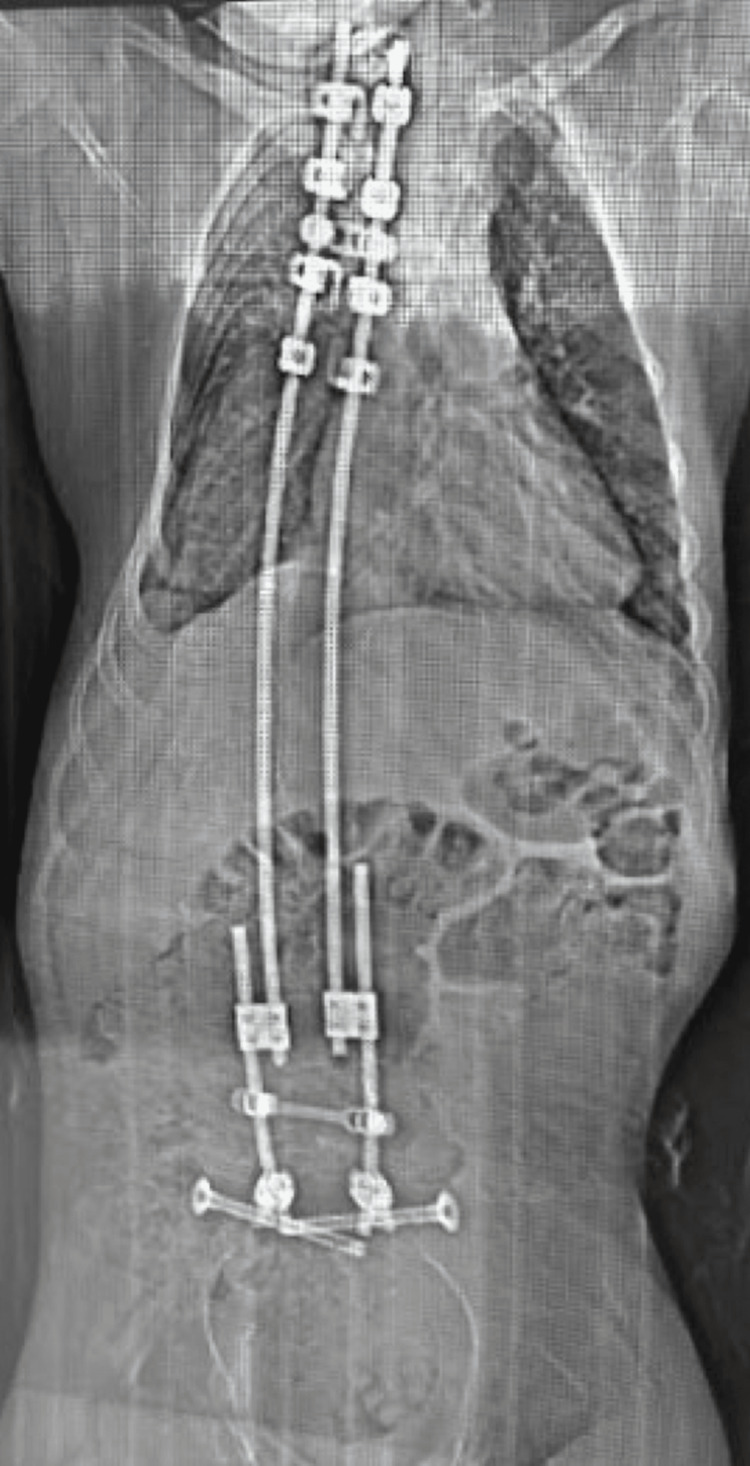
Latest follow-up AP full-spine radiograph depicting the final postoperative construct AP: Anteroposterior
